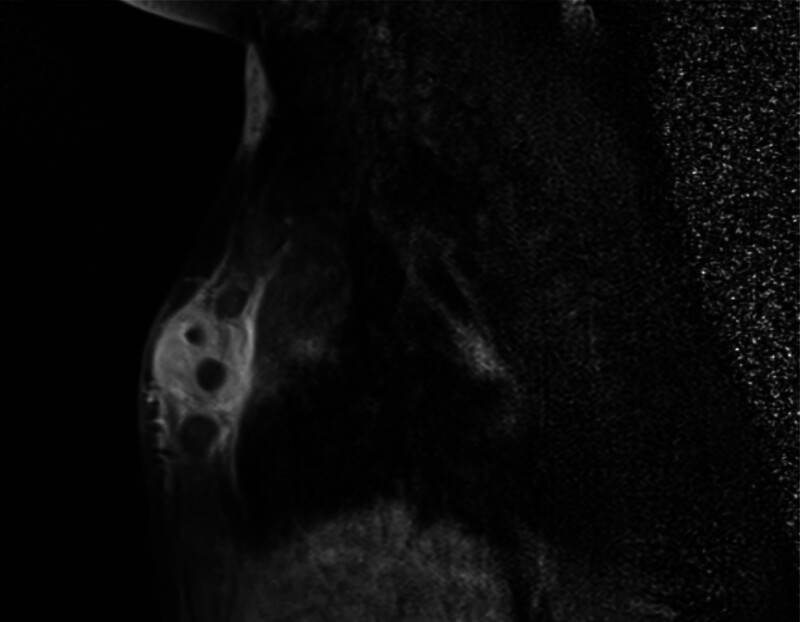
Sagittal MRI showin presternal mass reaching the retrosternal space, widening of gaps between sternal bony bodies.
